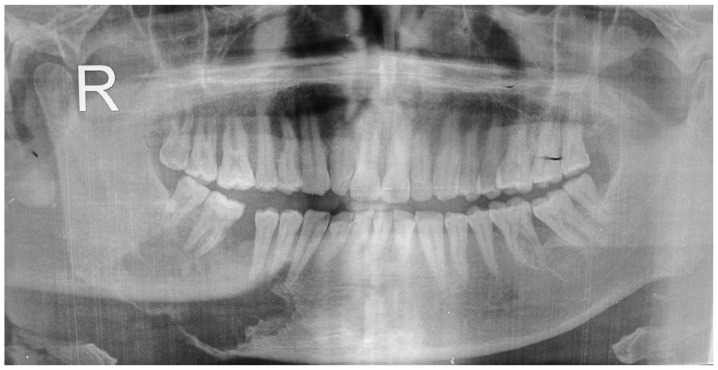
Initial panoramic radiograph showing a radiolucent lesion with a moth-eaten margin in the right mandible. The lesion resulted in the fracture of the mandible. The involved teeth appear to be floating on the lesion.
